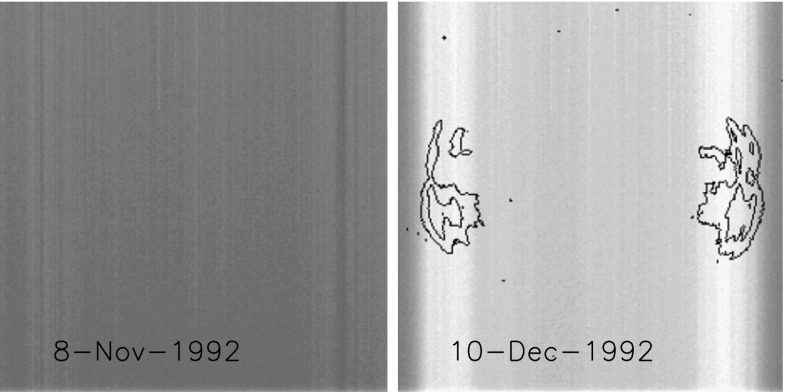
Change in QR 7.91 ms dark frame caused by the entrance filter failure of 13 November 1992. Dates of the two dark images are shown. The relative intensity scales of the two images have been adjusted to reveal the real change in dark signal. The contours in the right hand image are taken from Figure 26(B) and clearly demonstrate the spatial relation between areas damaged by ionizing (X-ray) radiation and increased spurious charge.
